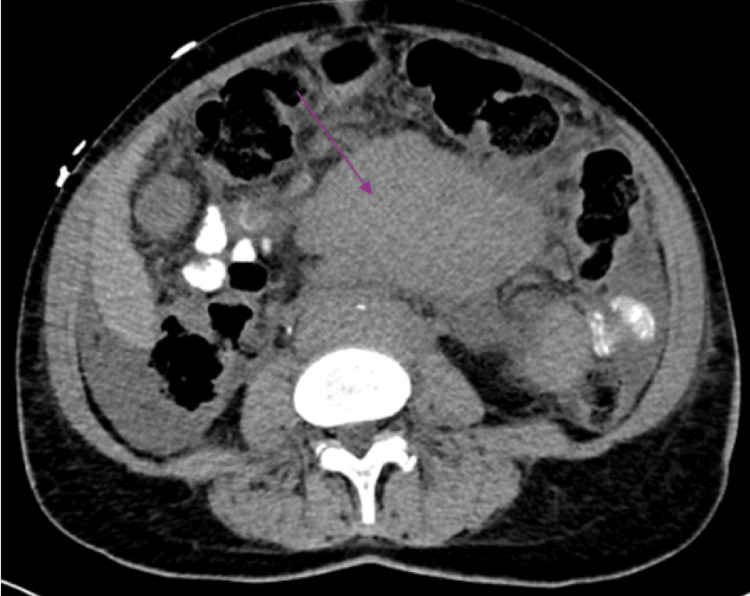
Contrast-enhanced axial CT image highlighting a bulky, heterogeneous abdominal mass (arrow) producing significant displacement of adjacent bowel structures.
